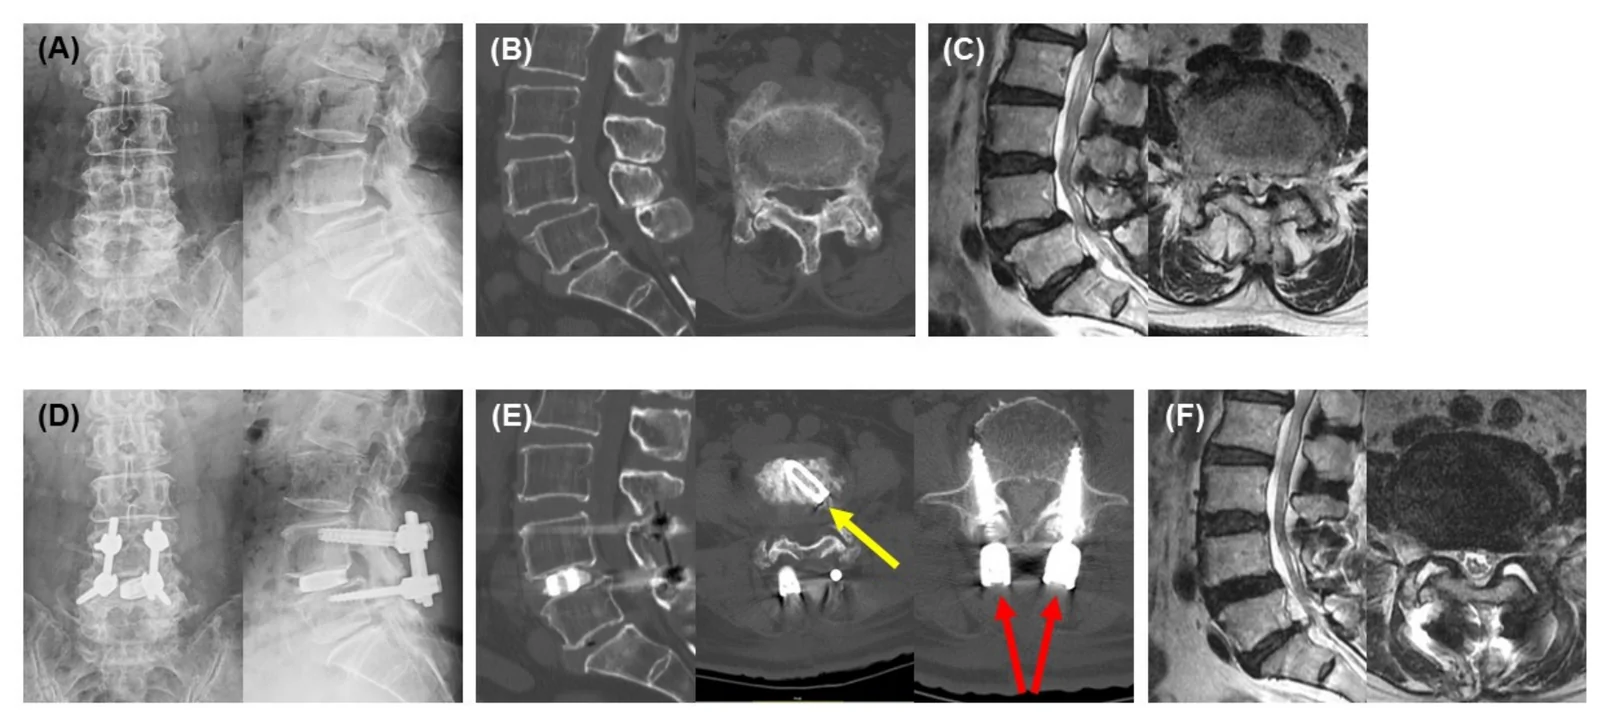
Representative case of transforaminal lumbar interbody fusion using cortical bone trajectory screws. (A) Preoperative X-ray images. (B) Preoperative computed tomography (CT) images. (C) Preoperative magnetic resonance (MR) images. (D) Postoperative X-ray images. (E) Postoperative CT images. Yellow arrow, cage insertion trajectory. Red arrows, screw insertion trajectory. (F) Postoperative MR images.
